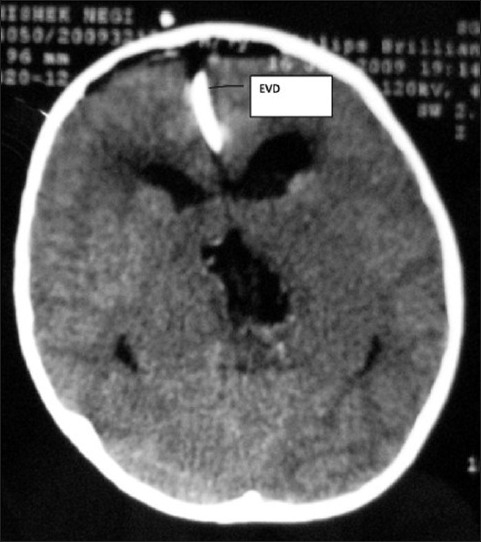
Axial section of noncontrast CT scan of head on postoperative day 1, showing external ventricular drain in situ with tumor cavity
